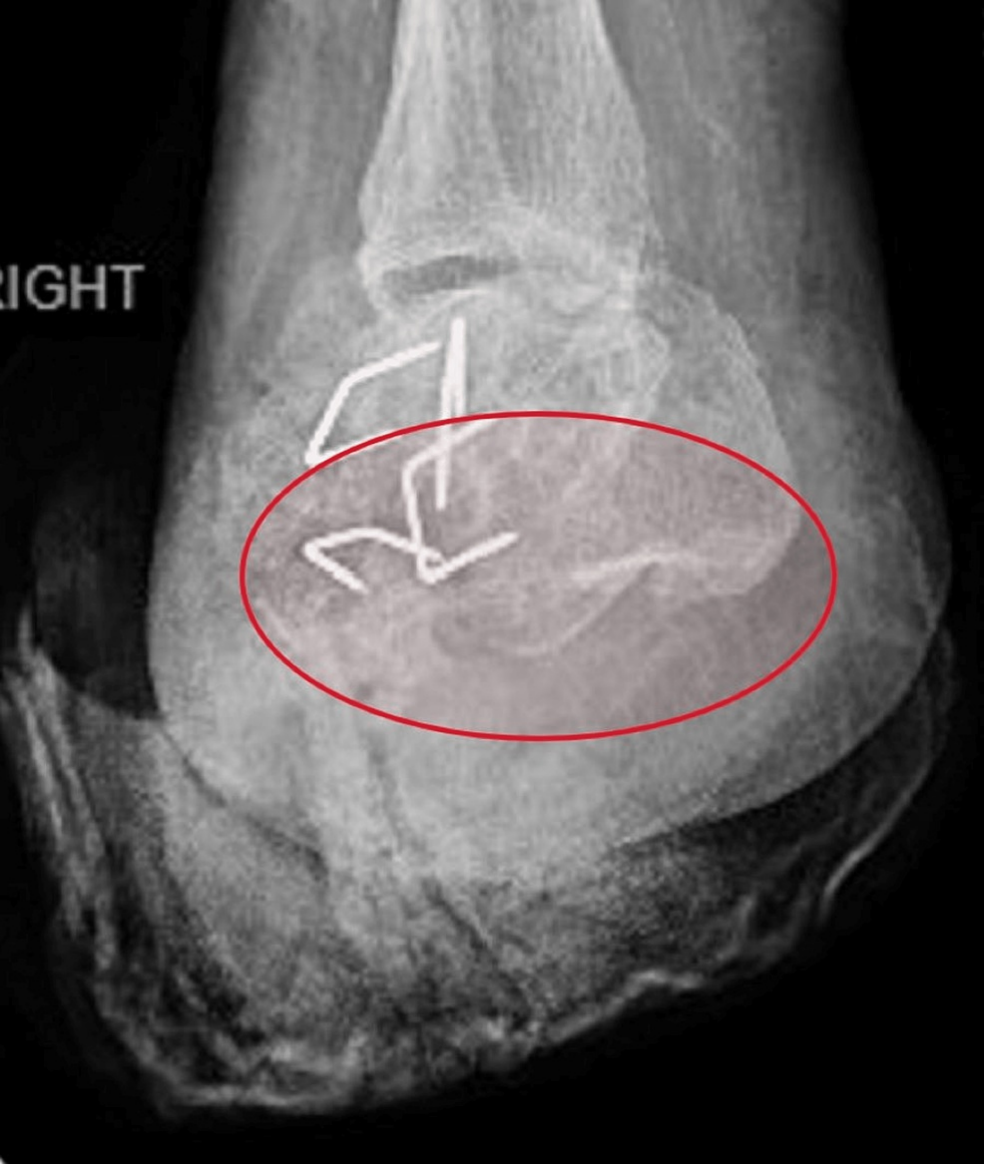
X-ray of the right foot and ankle showing postamputation changes of the hindfoot. The abnormal density of the remaining calcaneum and talus with erosive changes suggested the simultaneous presence of osteomyelitis.
